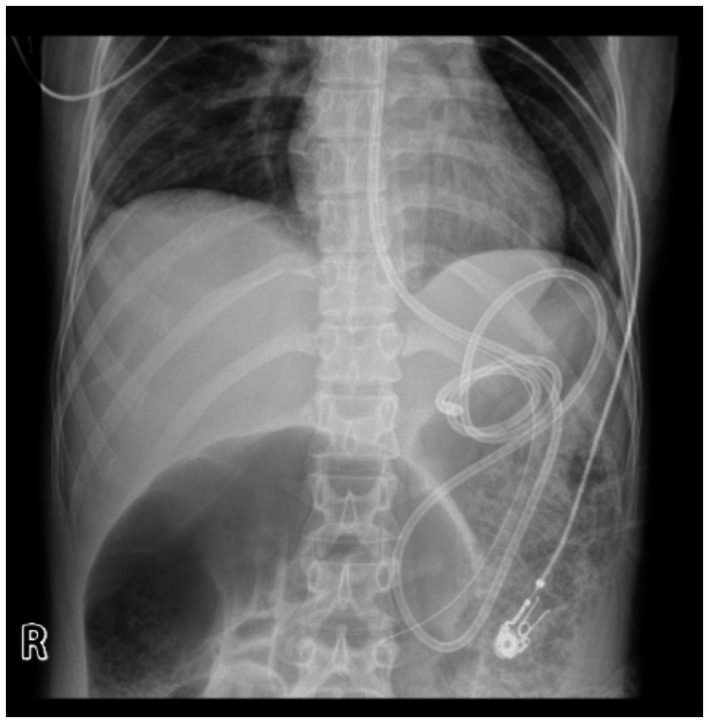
Abdominal X-ray. The small bowel gas accumulation and bowel dilation during gastrointestinal tube placement.
